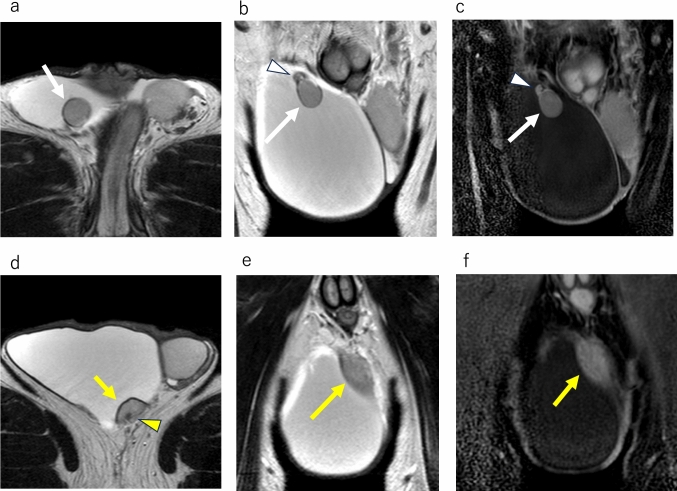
Polyorchidism in a 65-year-old male with supernumerary testis in right scrotum with hydrocele. a Axial T2-weighted MR image shows mild atrophied right testis (white arrow). b Coronal T2-weighted MR image shows the right testis (white arrow), attached epididymis (white arrowhead) with hydrocele. c Coronal contrast-enhanced fat-suppressed T1-weighted MR image shows enhancement of the epididymis (white arrowhead) and right testis (white arrow). d Axial T2-weighted MR image shows a mass with equal intensity to the left normal testis. Linear hypointense structure (yellow arrowhead) is observed in the mass. The mass is a supernumerary testis and the internal linear hypointense structure (yellow arrowhead) is the testicular mediastinum. e Coronal T2-weighted MR image shows a supernumerary testis (yellow arrow) with hydrocele. f Coronal contrast-enhanced fat-suppressed T1-weighted MR image shows enhancement of a supernumerary testis (yellow arrow)
